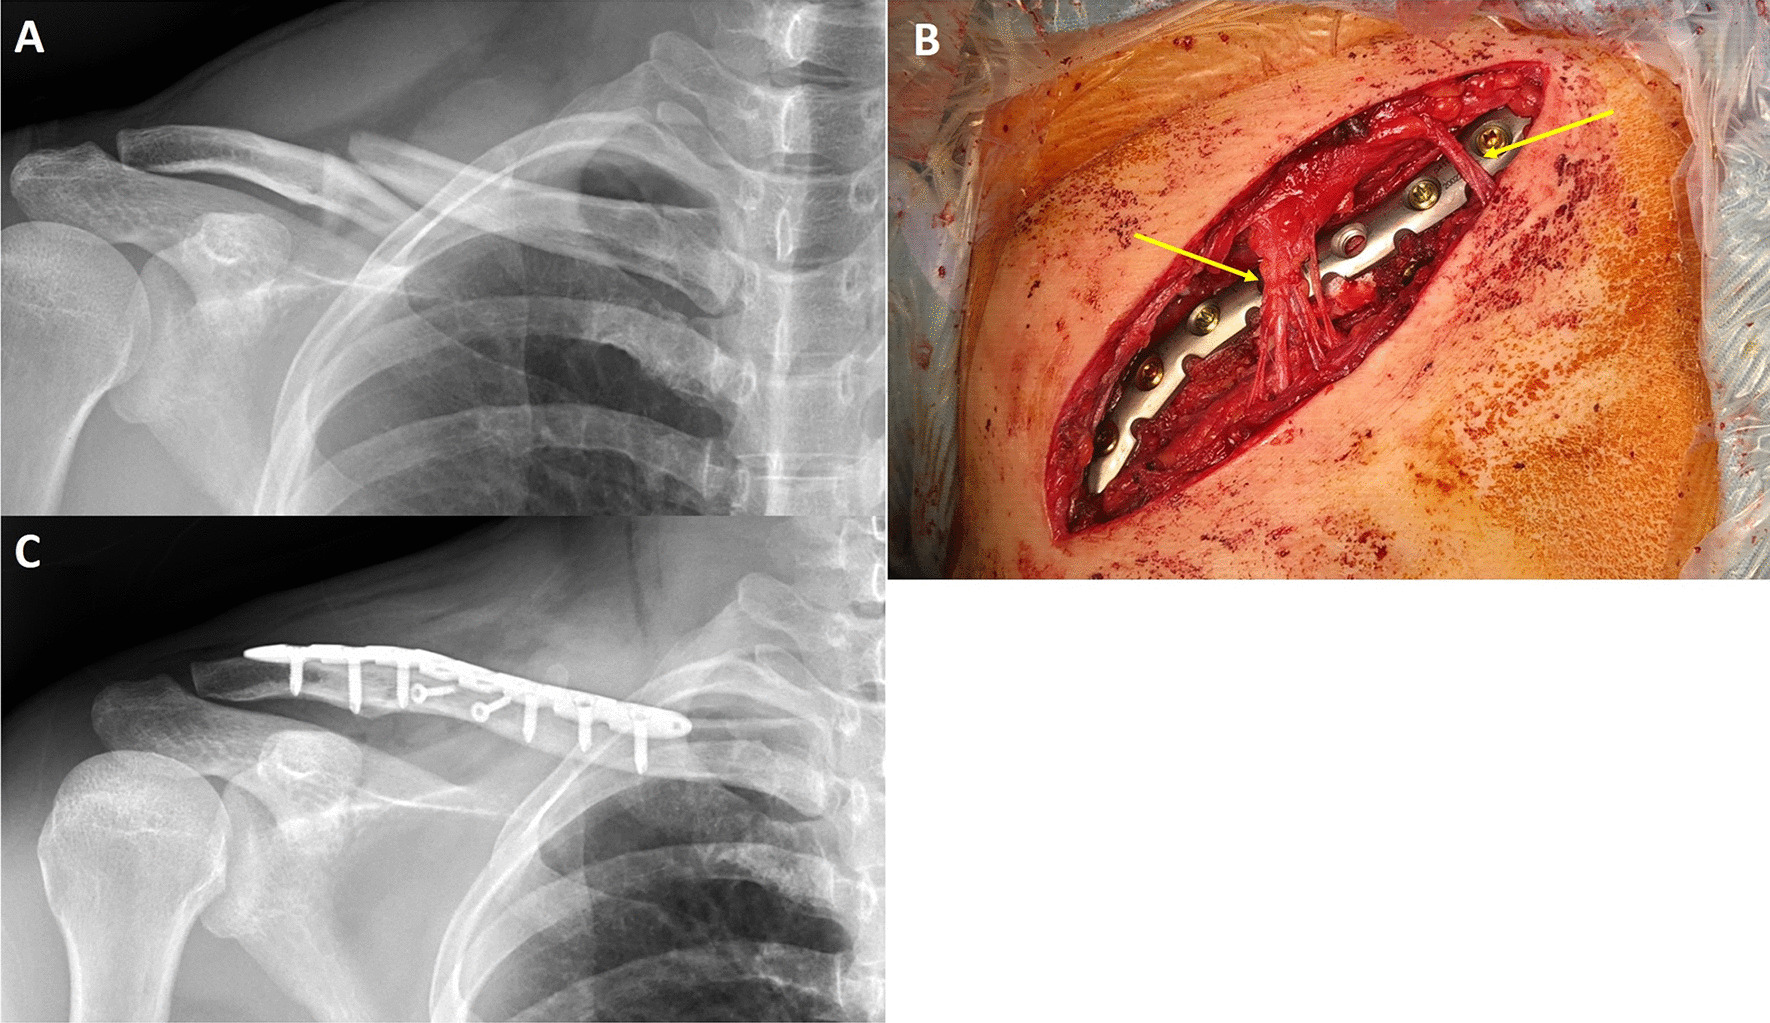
A case of right clavicle mid-shaft fracture after a motorcycle traffic accident, male, 34 years, AO/OTA classification 15.2B. a Preoperative radiograph showed right clavicle mid-shaft fracture with wedge fragment, displacement, shortening. b Intraoperative photograph, the 2 branches of supraclavicular nerve (yellow arrowhead) were preserved during operation. c Postoperative radiograph
